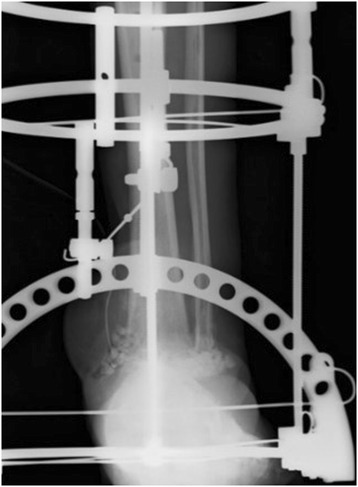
Anteroposterior plain radiography of the *left ankle*. An Ilizarov external fixator was applied after removing the internal fixation implant because of a deep infection
